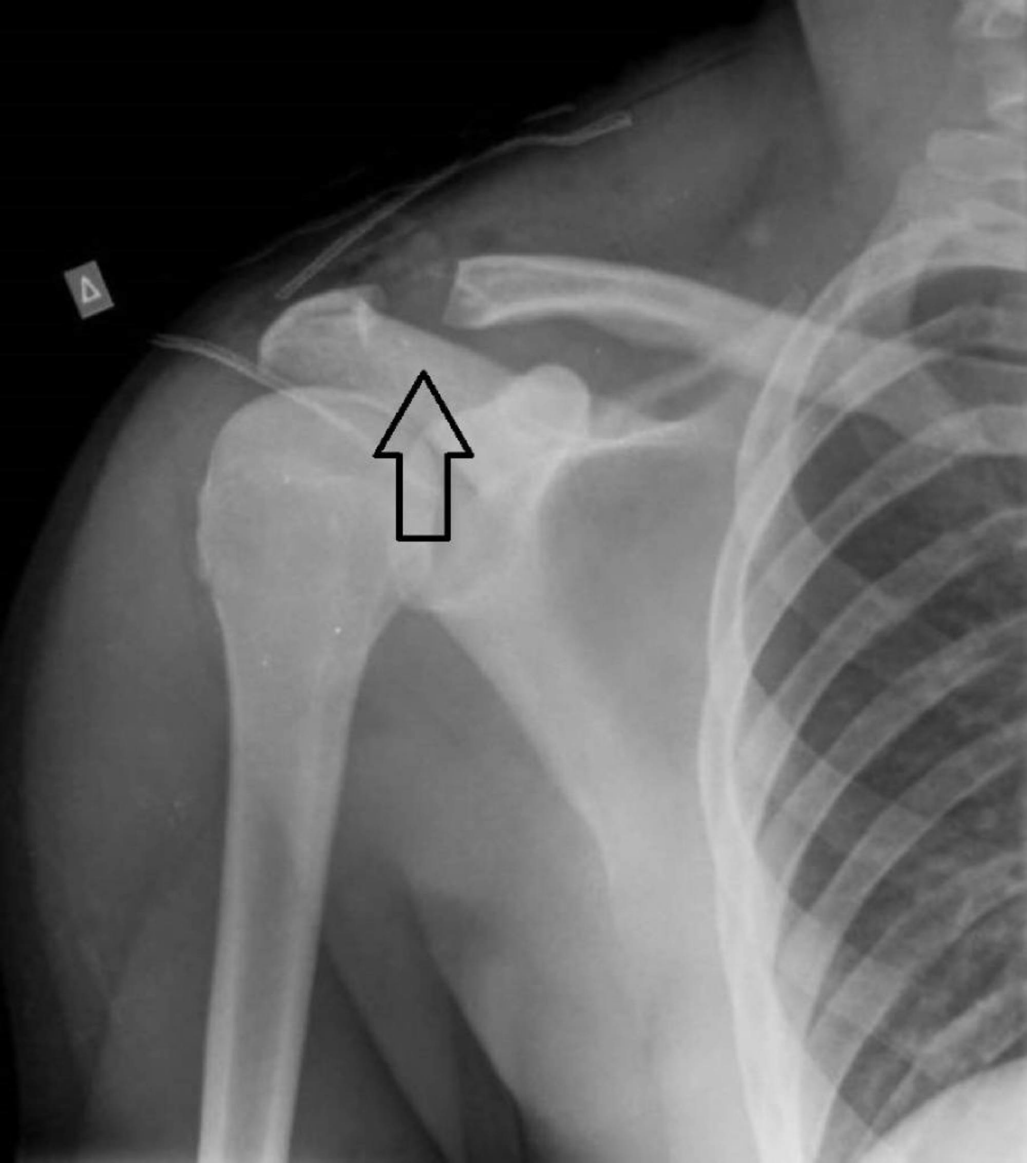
Postoperative anteroposterior radiograph. The tumor is fully excised.
